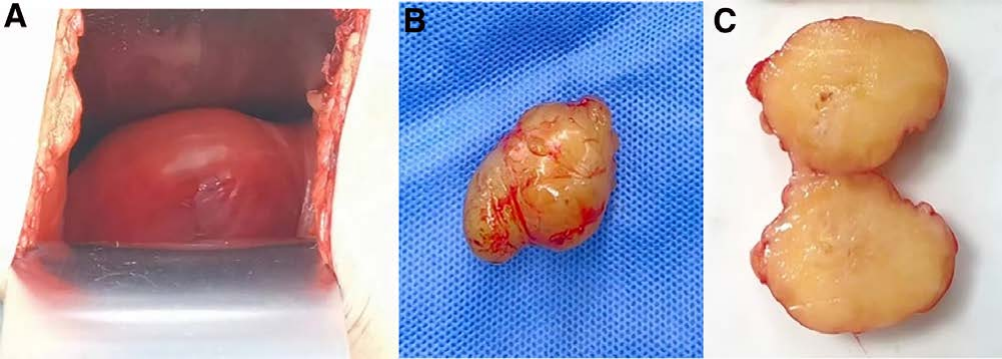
Gross (A,B) and longitudinal (C) view of the tumor. MRI = magnetic resonance imaging.
